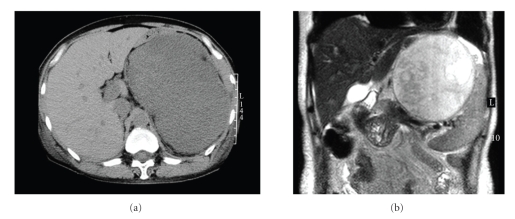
(a) Abdominal CT showing a huge tumor as a low-density mass occupying much of the left side of the abdominal cavity. (b) Sagittal T2-weighted MR image showing a mosaic-patterned high-intensity mass, with an enclosed low-intensity septum.
